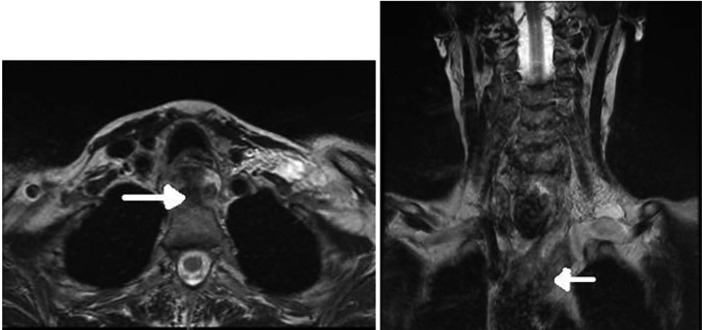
MRI neck with contrast at day 7. Residual hematoma is seen in the superior mediastinum and extending into the neck along fascial layers.
